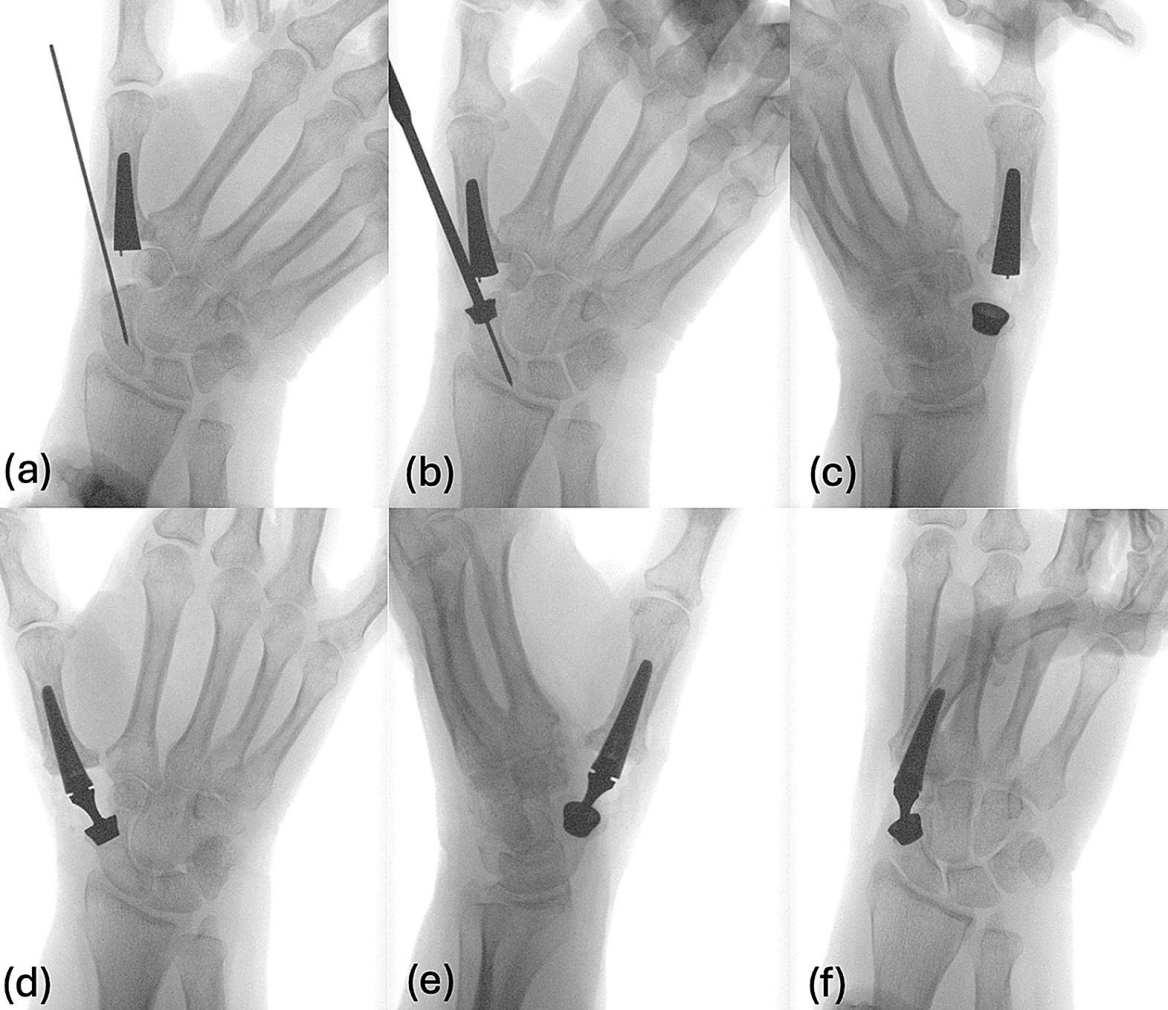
Individual surgical steps (a) shaft introduced in the first metacarpal bone. K-wire placed in the scaphoid. (b) pre-drilling of the scaphoid. (c) positioning of a conical cup in the scaphoid. (d) insertion of the neck. (e) X-ray in a second plane. (f) stress-test of the prosthesis with maximal opposition
